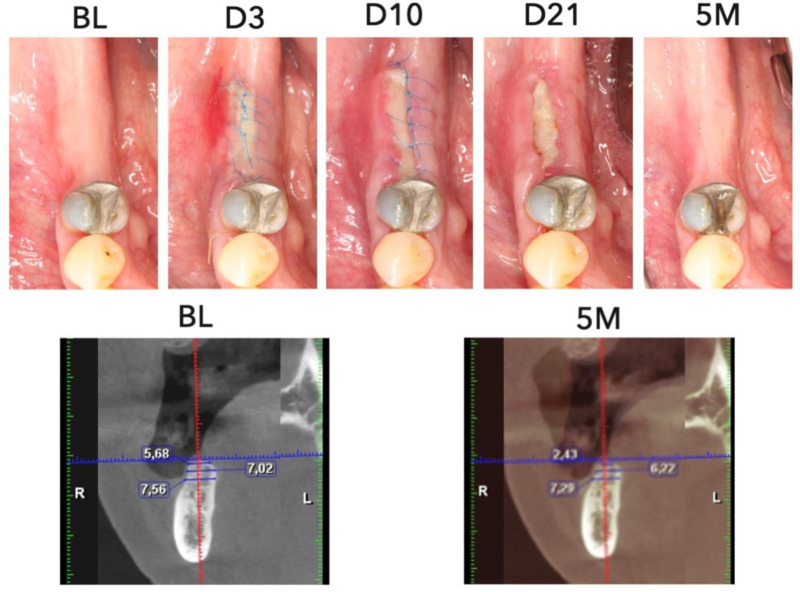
Case demonstration of compromised healing after guided bone regeneration procedure. Top row shows clinical intraoral photography from baseline (BL), 3 days (D3), 10 days (10D), 21 days (D21), and 5 months (5M). Bottom row shows CBCT bone width measurements at baseline (BL), 5 months (5M). There is evidence of inferior bone gain seen in clinical photos over time due to compromised healing and shown in bone loss measurements in the follow-up CBCT.
